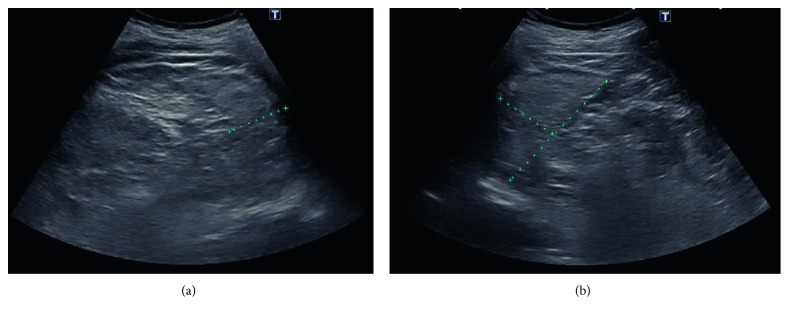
Splenic ultrasound. (a) Transverse dimension: 3.3 centimeters. (b) Long axis: 7.9 centimeters and anteroposterior: 3.9 centimeters. Atrophic spleen suggestive of multiple repeated episodes of infarction.
